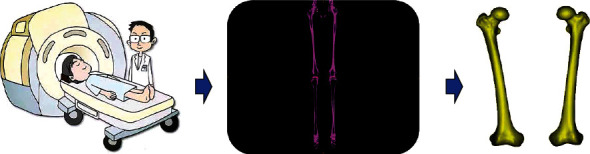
The process from CT scanning to 3D reconstruction of the femur.
